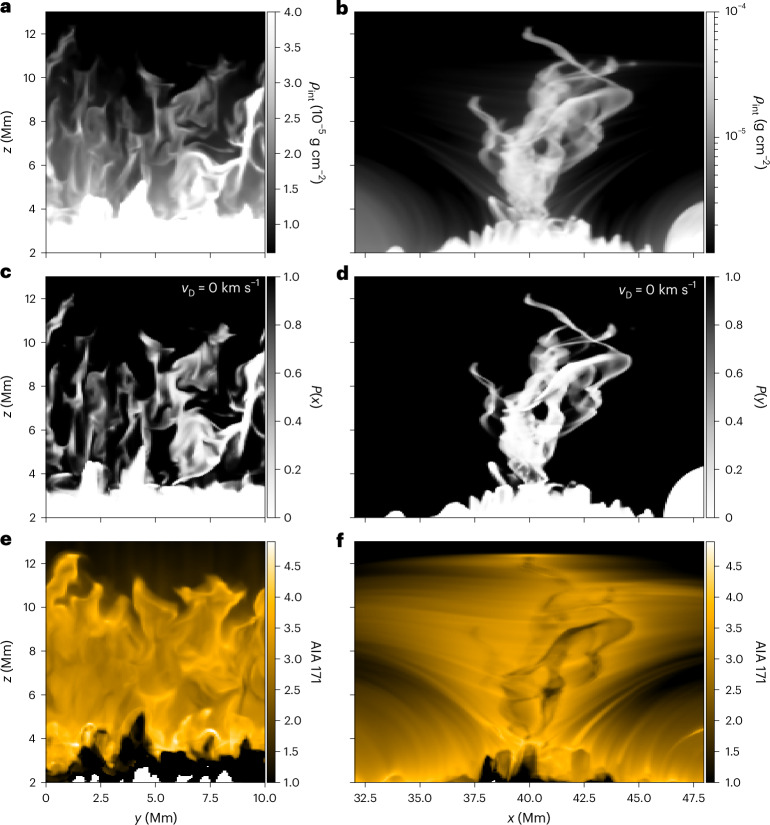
Appearance of the prominence in Run II NLTE in Hα and AIA 171. a–f, A comparison of integrated density (a and b), Hα emission in the line core (c and d) and AIA 171 emission (e and f) for the prominence view in Run II. a, c and e show the prominence from the side (line-of-sight along the *x* axis), and b, d and f show the prominence from the front (line-of-sight along the *y* axis). Following ref. ^48^, the Hα emission is approximated by calculating an integrated hydrogen opacity that uses the non-equilibrium hydrogen populations from the NLTE version of the MURaM code. The shown emission is taken in the Hα line core (that is, at a Doppler velocity of *v*_D_ = 0). The AIA 171 emission is calculated following ref. ^49^ and takes absorption into account. Extended Data Fig. 6 equivalently shows the appearance of the prominence in Run I NLTE.
